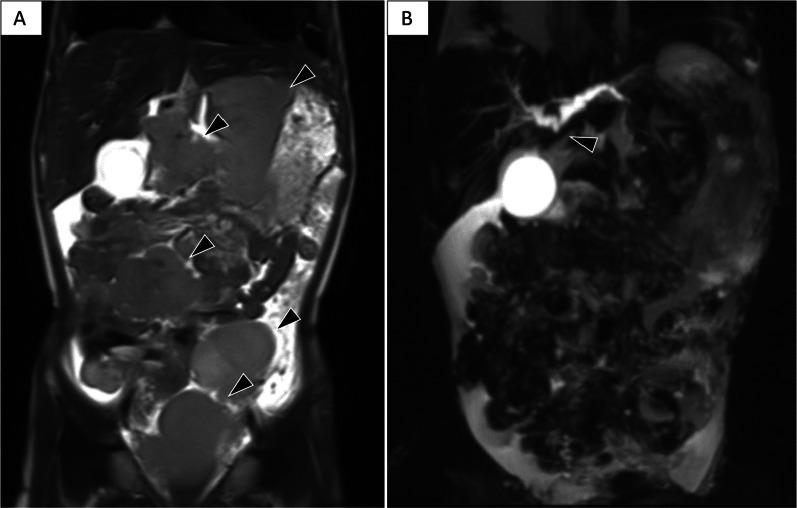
Magnetic resonance imaging at initial examination. A T2-weighted image shows multiple tumors occupying the abdominal cavity (arrowhead). B Maximum intensity projection image showing biliary obstruction at the level of the common hepatic duct (arrowhead)
